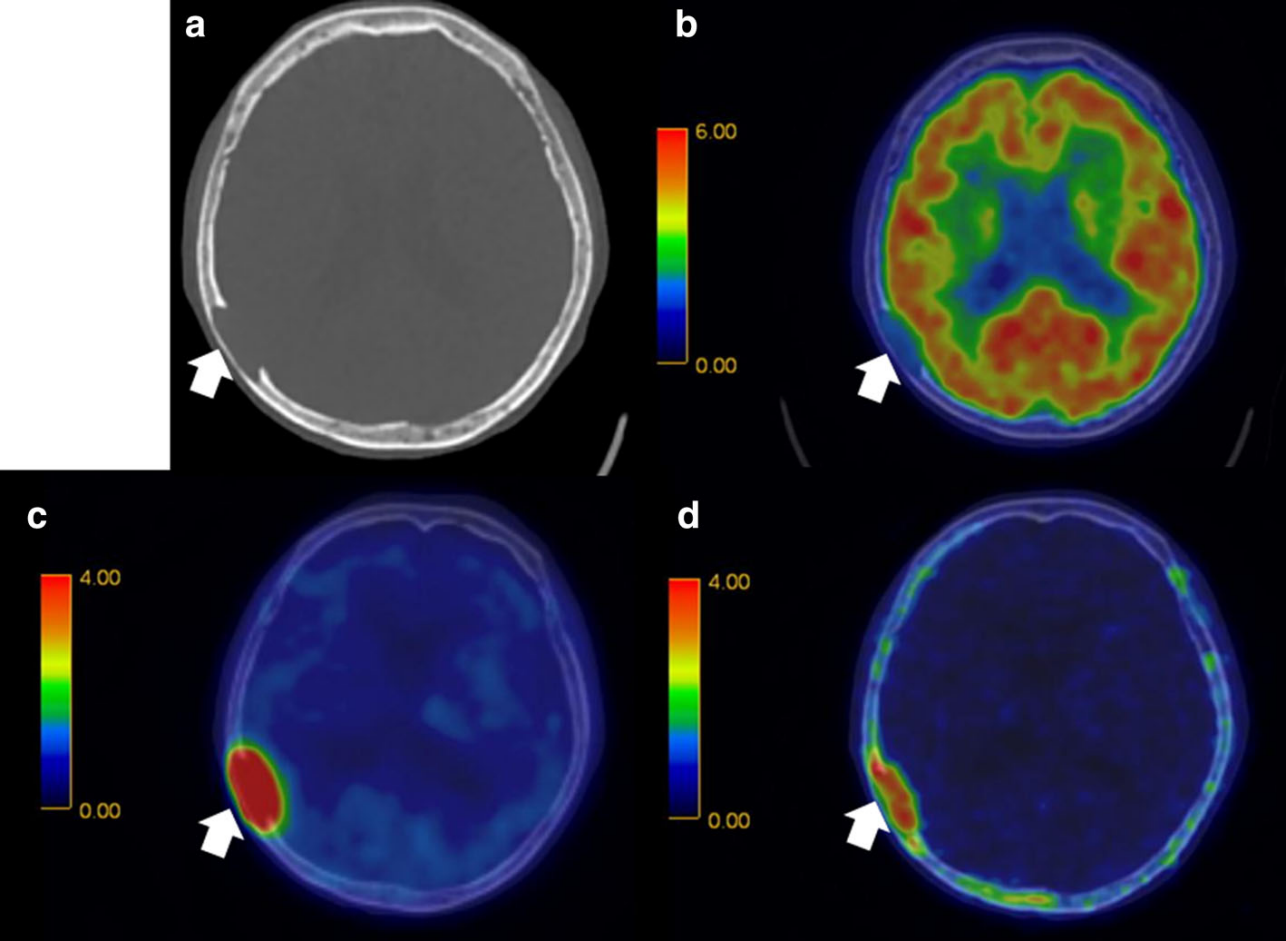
Fusion images of a CT, b
